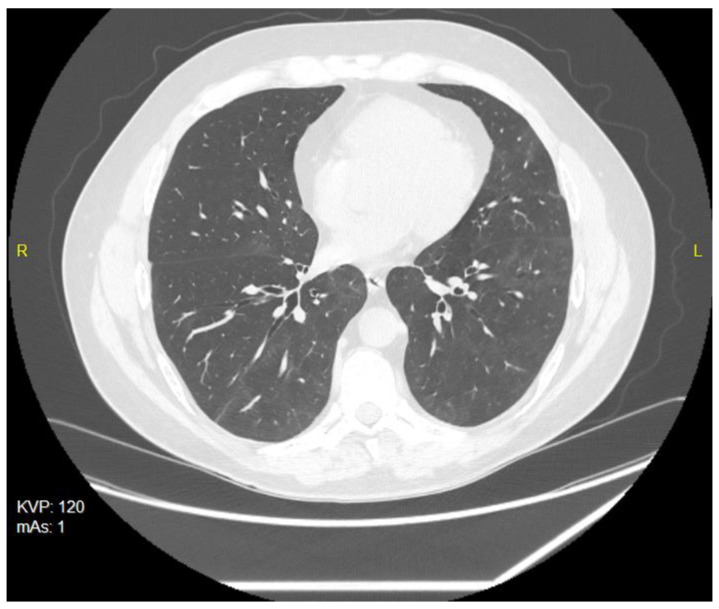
CT scan of the chest of 20 February 2021—small, bilateral infiltrates of the grain-glass opacity type.
